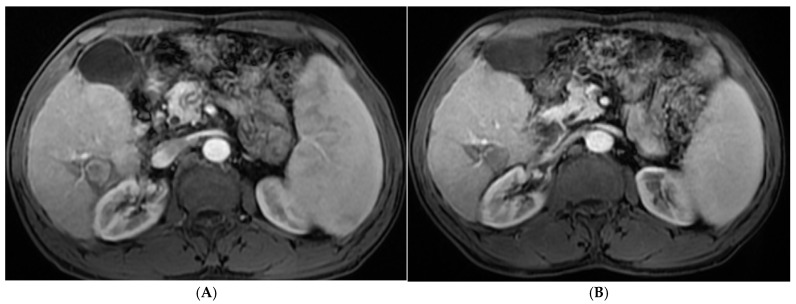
(A). Triphasic MRI abdomen (arterial phase) axial cuts revealing right lobe segment VI focal lesion with homogeneous enhancement (before RFA). (B). The same lesion (arterial phase) axial cuts one month after RFA.
